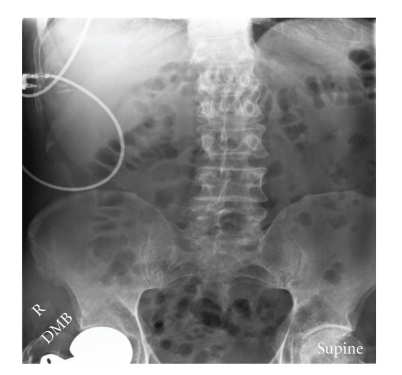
Pelvic/abdominal X-ray showing osteopenia.
